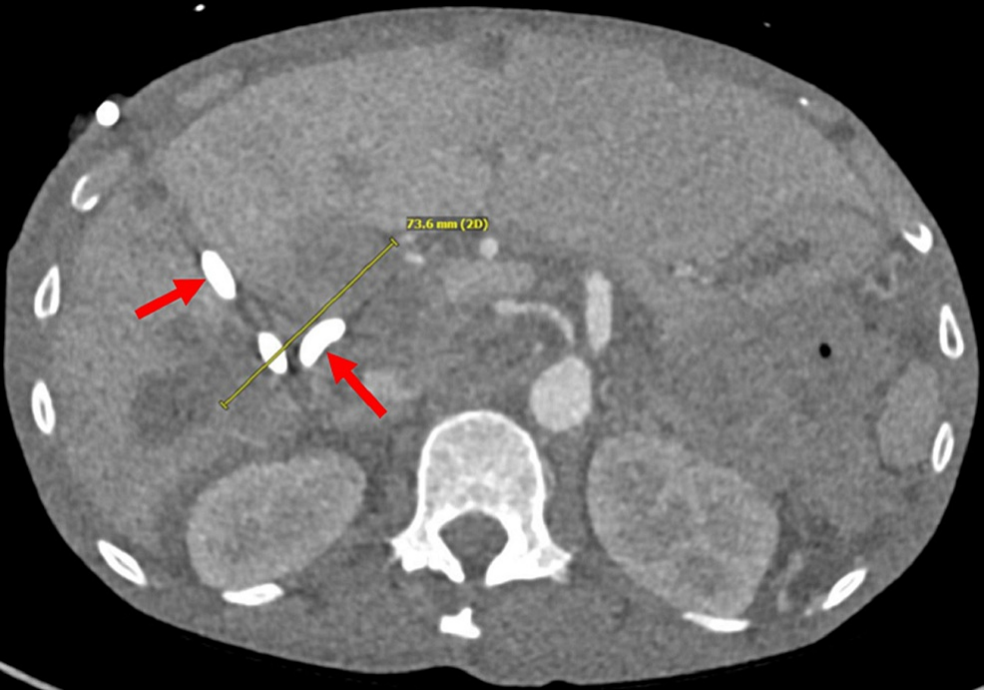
Pigtail Catheter Placed Within the Intrahepatic Abscess A post-procedural axial computed tomography at the level of the inferior right hepatic lobe demonstrates the percutaneous 10 French pigtail drainage catheter (red arrows) centered within the intrahepatic abscess (yellow measure).
